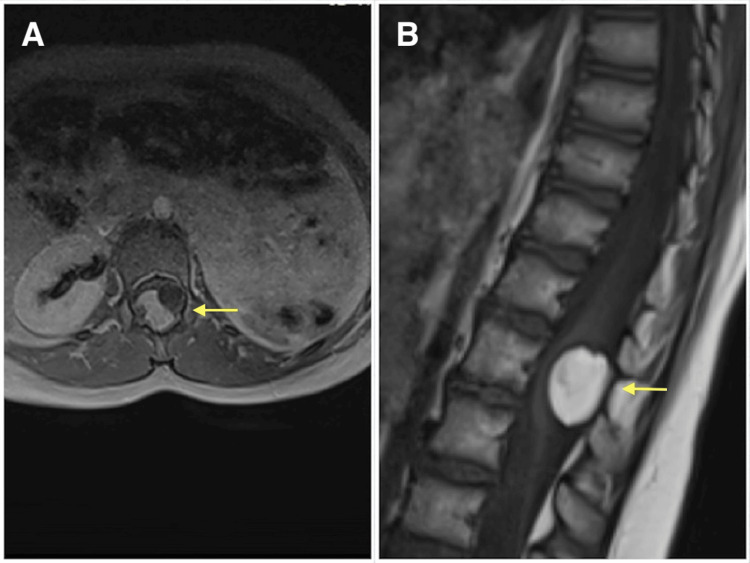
Axial (A) and sagittal (B) T1-weighted MRI scans of the spine showing a well-defined, hyperintense, intradural lesion with a nodular hypointense component at L1 (yellow arrow).
